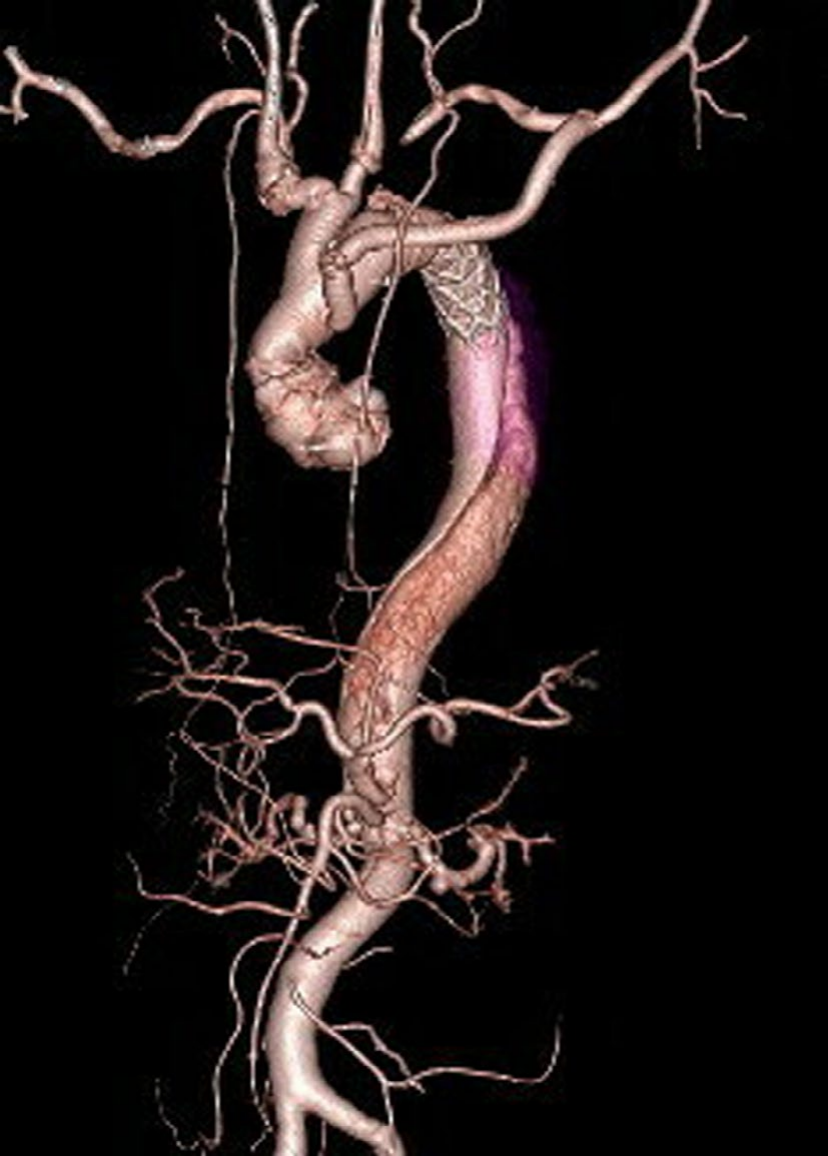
Post‐operative 3D computed tomography imaging showing ascending aorta and aortic arch replaced with an artificial graft
